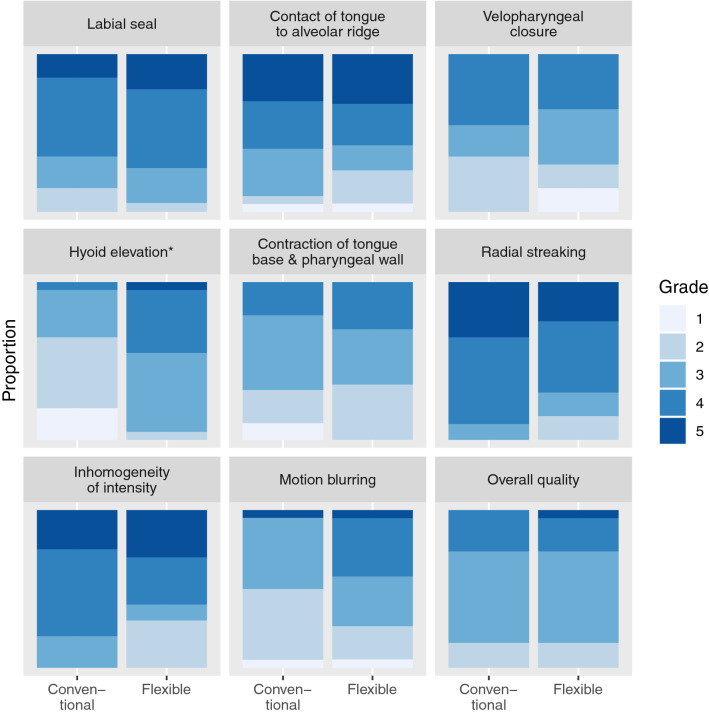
The proportions of grades given by the reviewers for the nine questions about the real-time MRI of swallowing. For this figure only, the grades for the five different subjects and by the four reviewers have been pooled. The grade 5 related to the best image quality or least artefacts, while 1 related to the worst image quality or most artefacts. For the visualisation of hyoid elevation, the odds of receiving a better grade was significantly higher for the flexible coil (*P* = 0.029) than for the conventional coil. For the other aspects of real-time MRI of swallowing, no differences in odds were found
